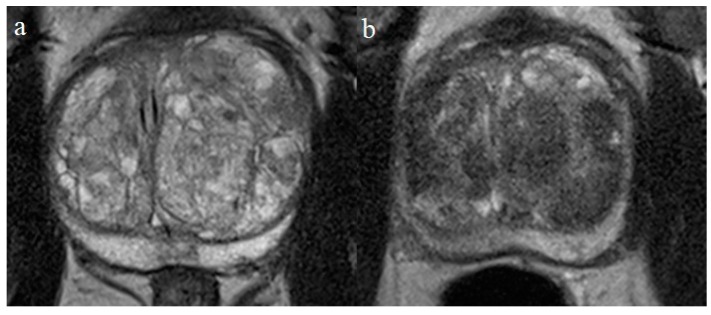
(a) Benign prostatic hyperplasia in a patient demonstrated on magnetic resonance imaging with T2-weighted turbo spin echo sequence in the transversal plane. Notice the adenoma in the left lobe compressing the prostatic urethra; (b) the same patient three months after treatment with a reduced T2 signal corresponding to infarction of the adenoma.
